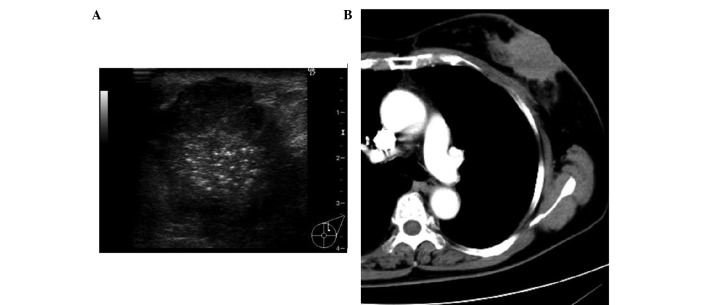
Diagnostic imaging. (A) Ultrasound imaging identified a lobulated, solid tumor measuring ≥3 cm of the left mammary gland. (B) Computed tomography of the chest indicated a mass lesion in the apocrine carcinoma region of the left mammary duct exhibiting heterogeneous and moderately enhanced microcalcifications.
